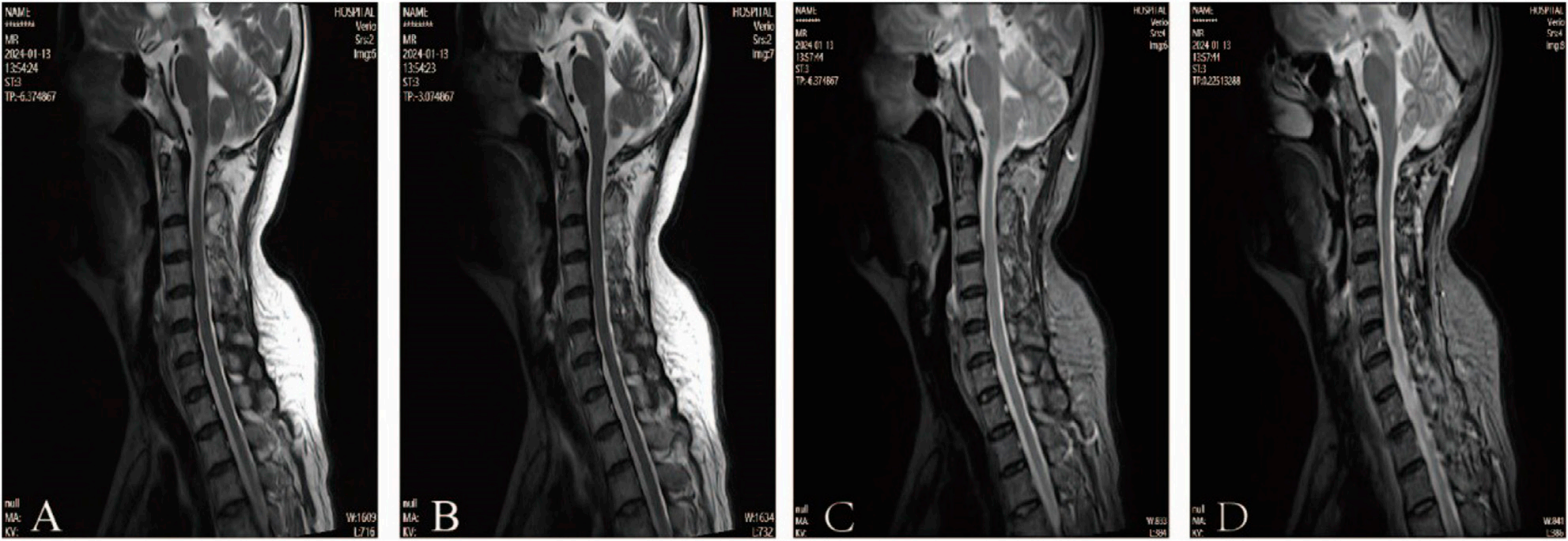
Magnetic resonance imagings of the cervical cord in July, 2023 (A–D).
